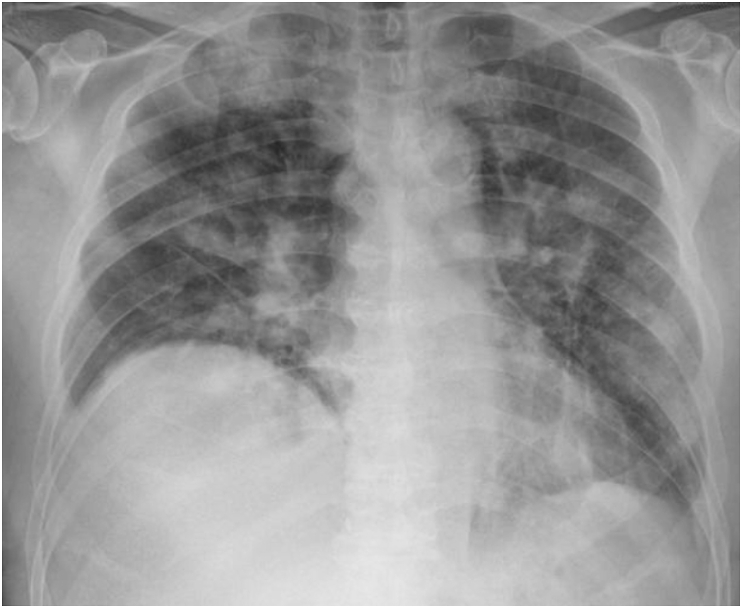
Chest radiograph of Case 2 on day of admission which shows bilateral multifocal airspace opacities.
